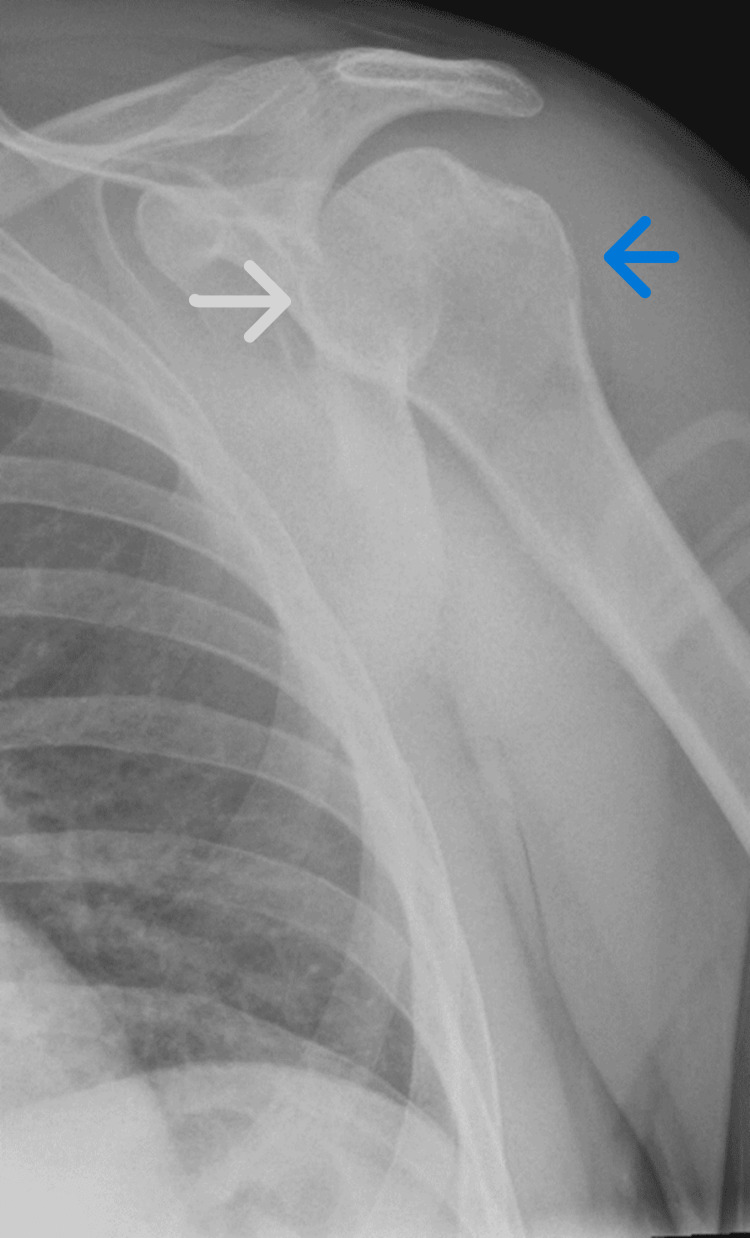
X-ray demonstrating successful reduction of the anterior dislocation Normal relationship now seen between the humeral head (blue arrow) and the glenoid fossa (white arrow).
